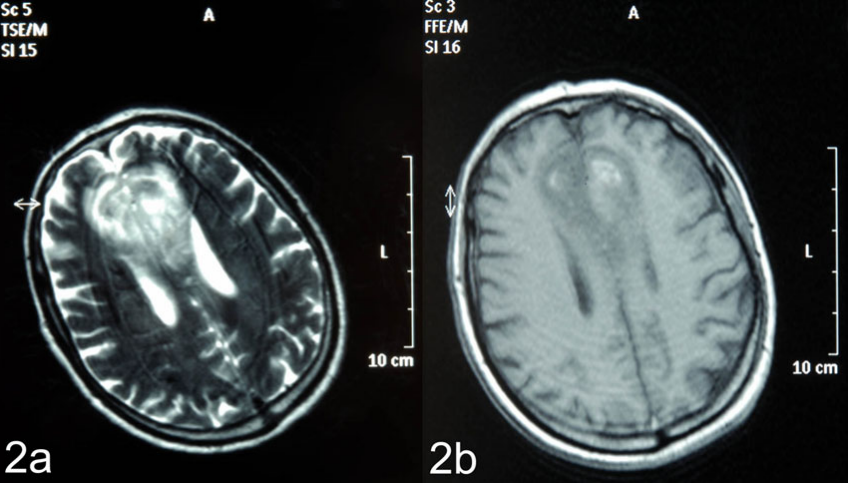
T1/T2 weighted magnetic resonance images initially erroneously reported as demonstrating a bifrontal neoplastic space-occupying lesion.
